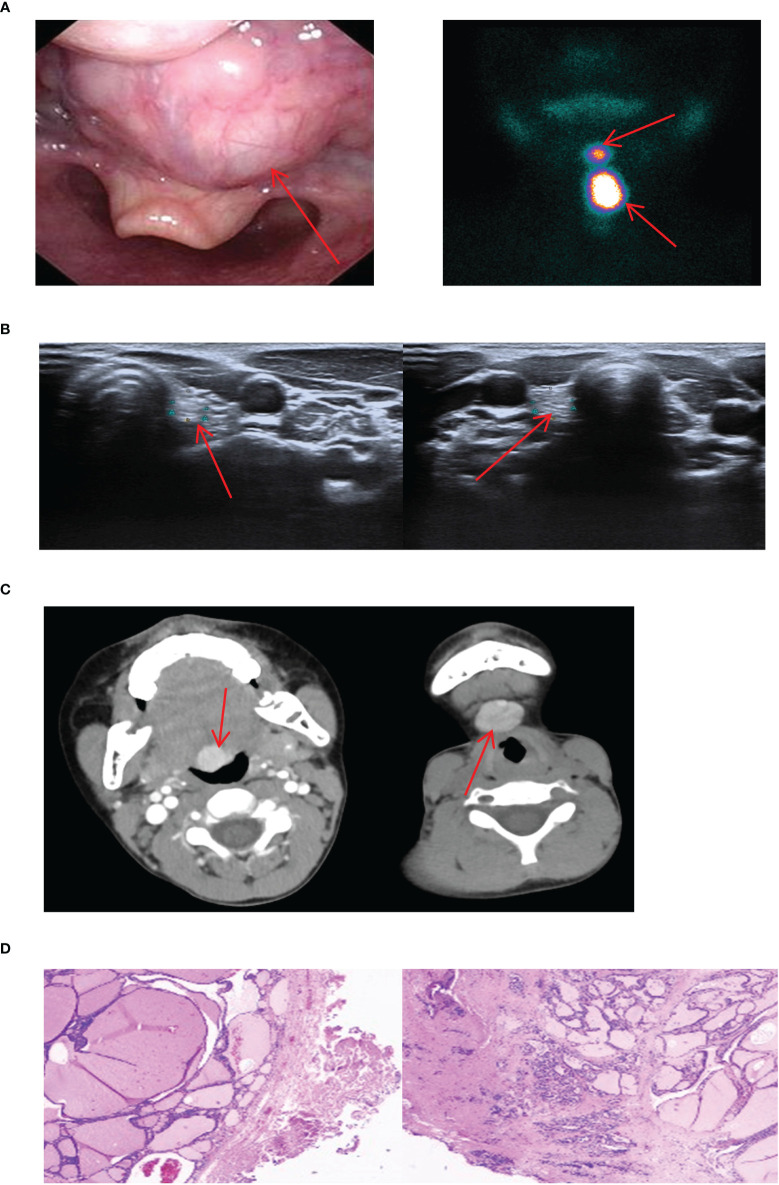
Imaging manifestations and pathological findings of the lingual + submandibular dual ETG. (A): The laryngoscope showed a large, reddish, round new creature at the root of the tongue with a smooth surface and mobility. Static imaging of thyroid gland respectively showed a circular abnormal aggregation in the distribution of radioactivity at the root of the tongue and the submandibular position. (B): The thyroid ultrasound showed the bilateral small thyroid glands. (C): CT respectively showed a circular mass with clear boundaries at the root of the tongue and the submandibular position. (D): The postoperative pathology of the lingual + submandibular dual ETG both showed the nodular goiters.
